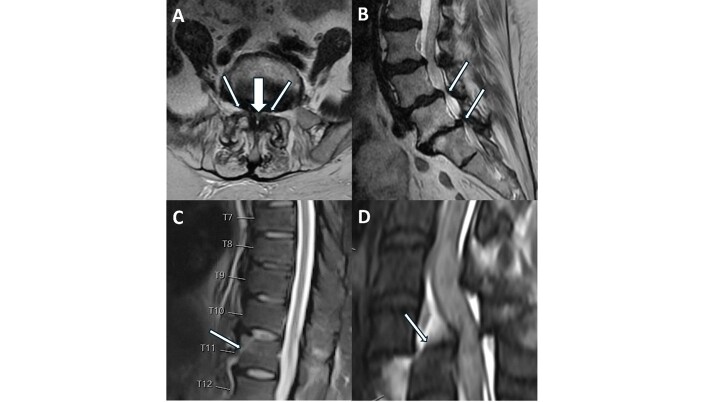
Representative positive findings in STAT magnetic resonance imaging of the spine. A: Severe spinal canal (thick arrow) and bilateral subarticular zone (thin arrows) stenosis. B: Severe spinal canal stenosis at L4–5 and L5–S1. C: Thoracic spine distraction fracture without canal stenosis. D: Cervical spine translation fracture with ligamentous and disc injury with cord edema and hemorrhage.
